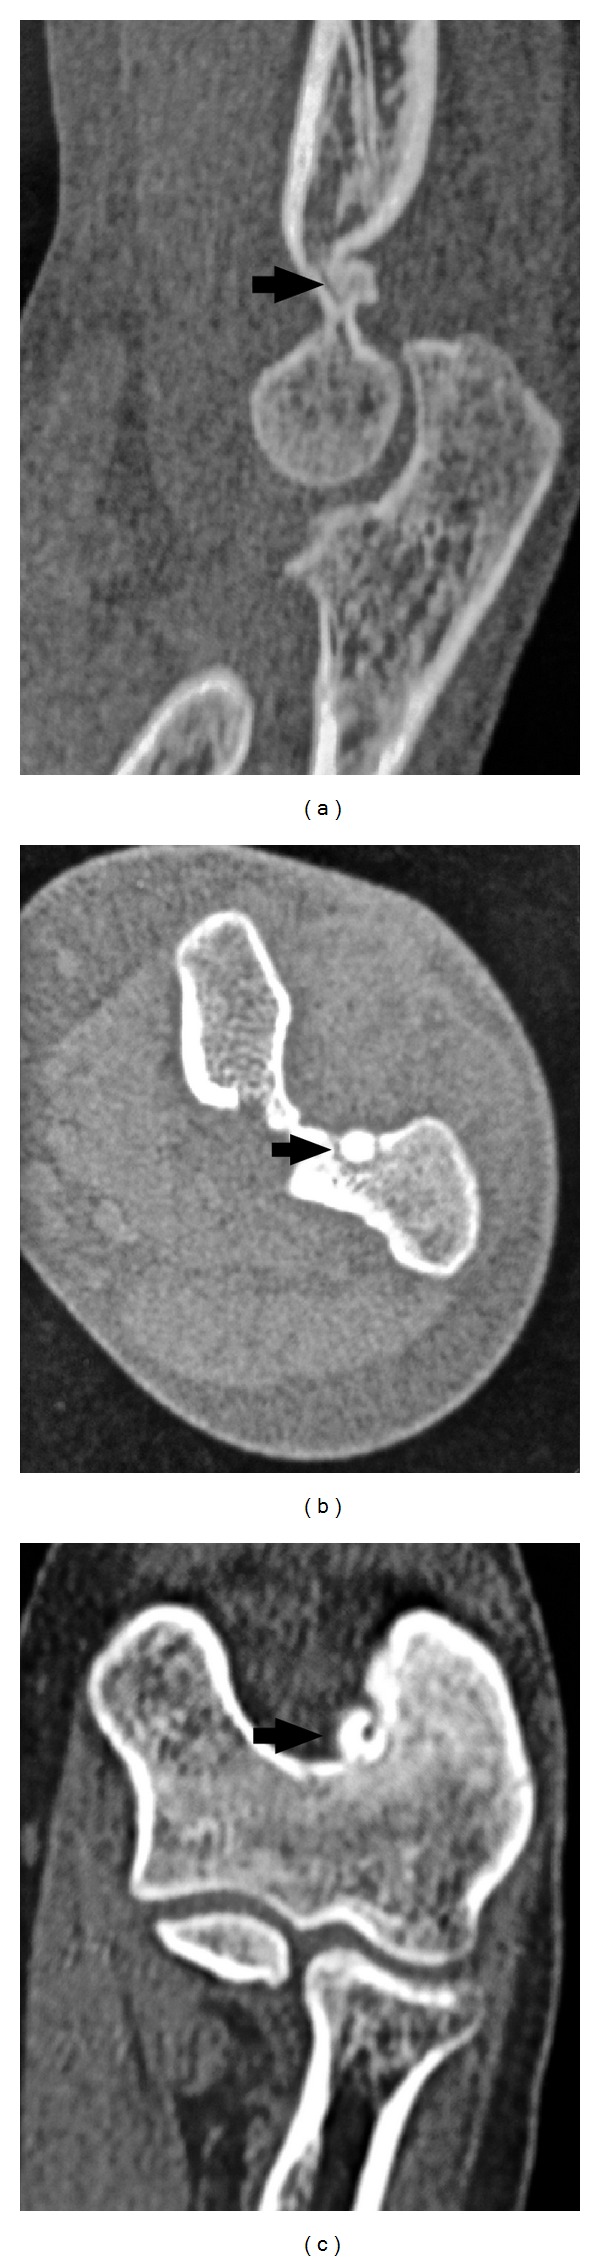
Sagittal, axial, and coronal computed tomography sections of the elbow show a 7 mm diameter cystic lesion that respects the cortical bone and is located at the cartilage-cortical bone junction in the posterolateral part of the olecranon fossa.
